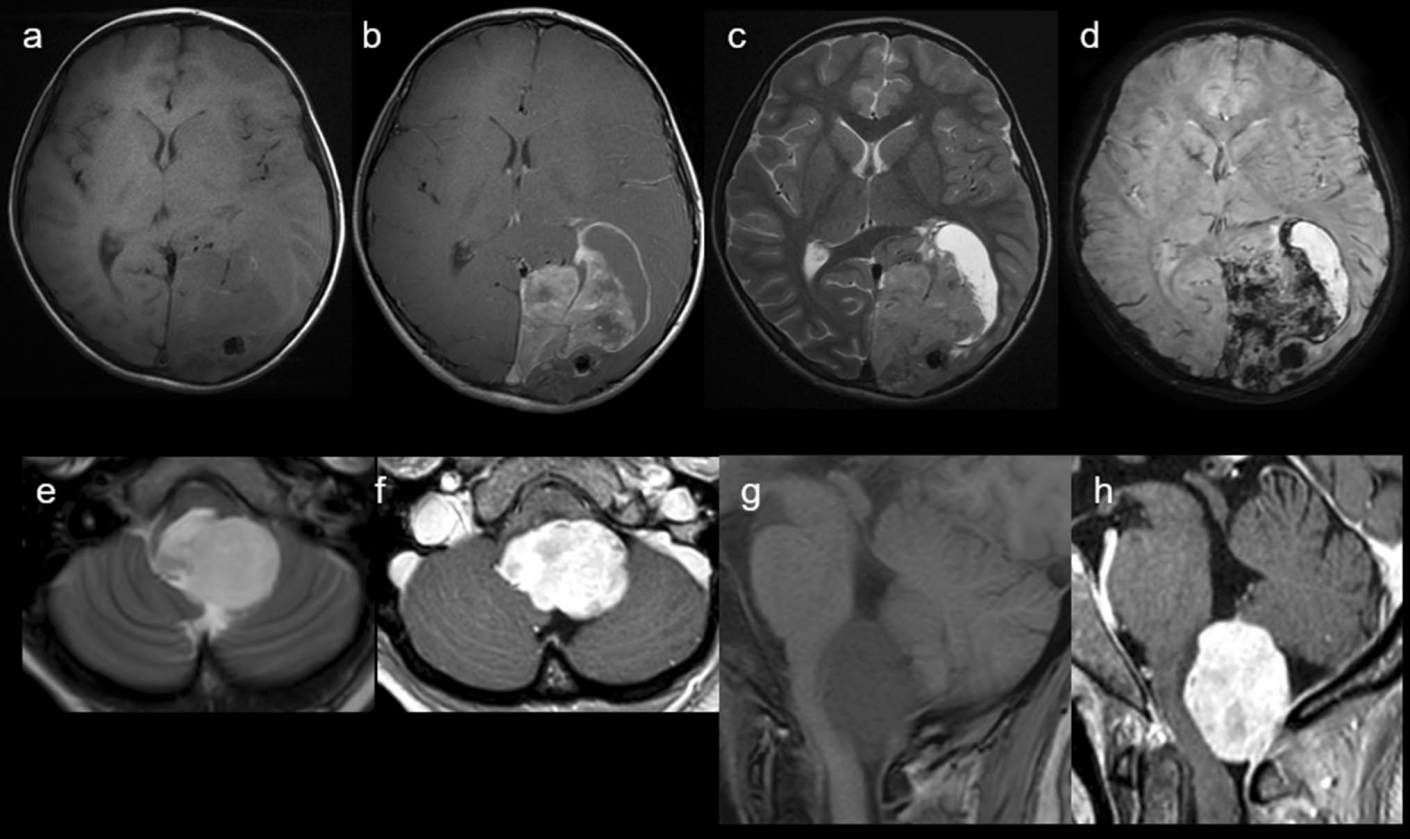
Radiological features of #cases 1 and 2. Case#1 (a–d): a MR images show a left occipital mass with tissue and cysts, low signal intensity on T1-weighted images, and strong contrast enhancement (b), heterogeneous high signal intensity on T2-weighted images (c) and extended hemorrhage on susceptibility-weighted images (d). Case#2 (e–h): e–g MR images show a mass dorsally exophytic from the medulla oblongata, with high signal intensity on T2-weigthed images, low signal intensity on T1-weigthed images (f–h) and very strong and homogeneous contrast enhancement. *MR* magnetic resonance
